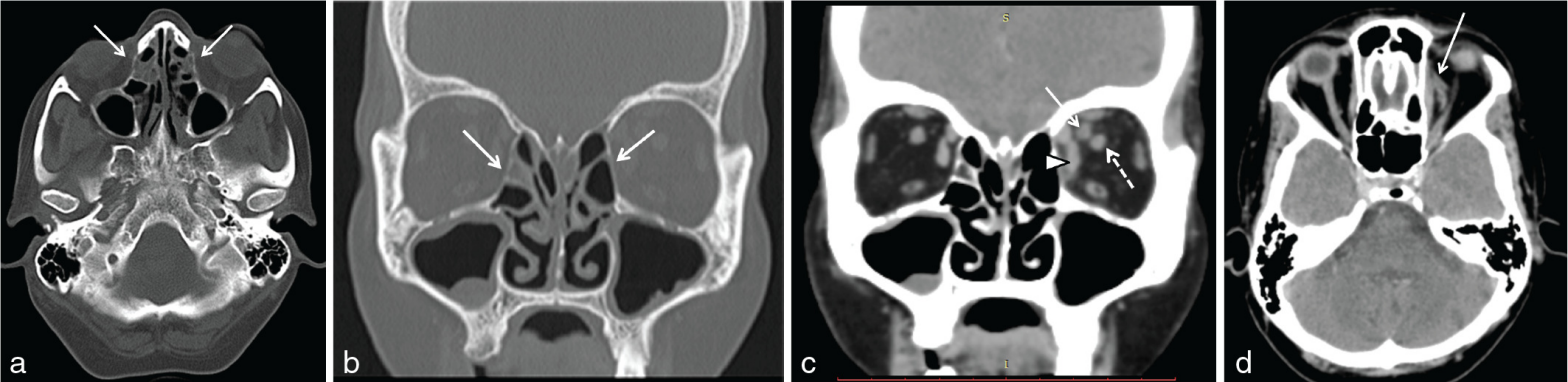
(a, b) Axial and coronal plain CT scans in bone window showing mucosal thickening of the bilateral ethmoidal and maxillary sinuses (arrows). (c, d) Contrast-enhanced CT scan in the coronal and axial sections showing linear non-enhancing soft tissue (arrows) located superomedial to the optic nerve (broken arrow). The thickening of the medial rectus muscle and the surrounding fat stranding (arrowhead) is significant.
